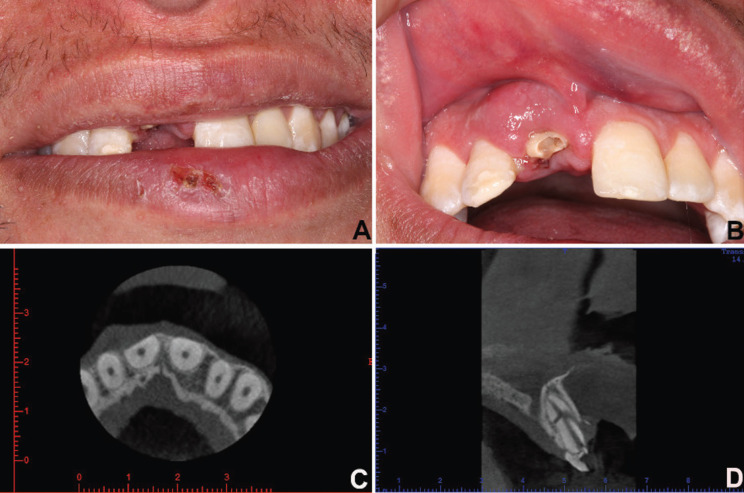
A) Abrasions and localized labial edema. B) Gingival edema and clinical crown fracture apparent without pulp involvement in teeth 11. clinical insertion. A cone beam computed tomography examination showed C) bone fractures and D) multiple root fractures.
